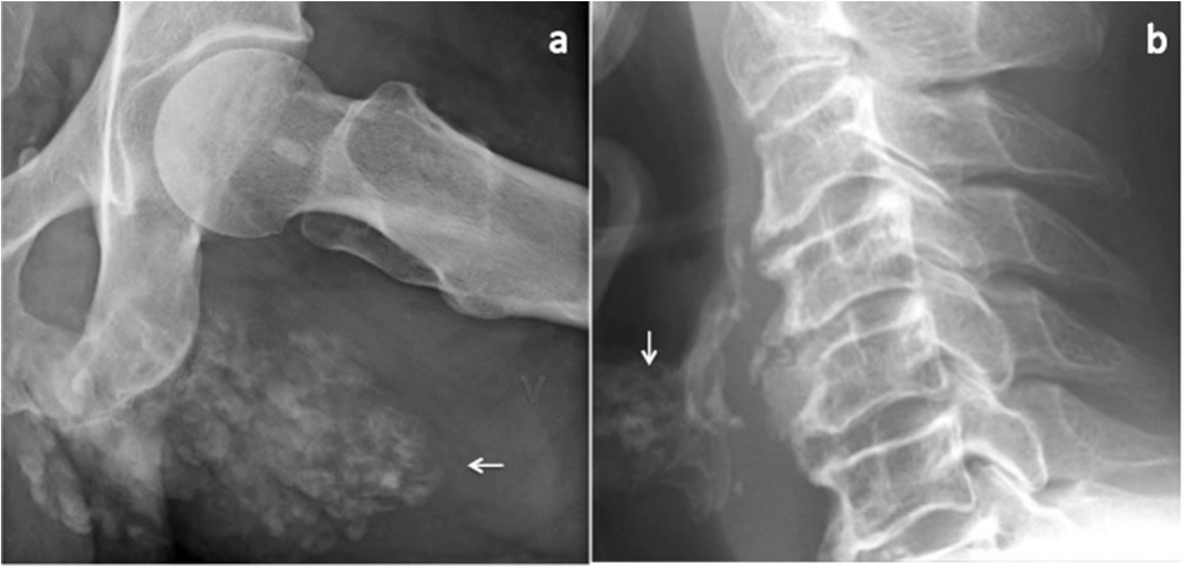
Radiographic findings. The pictures show a) the left gluteal calcification in subject 1, and b) age-inappropriate thyroid cartilage calcifications in subject 2.
